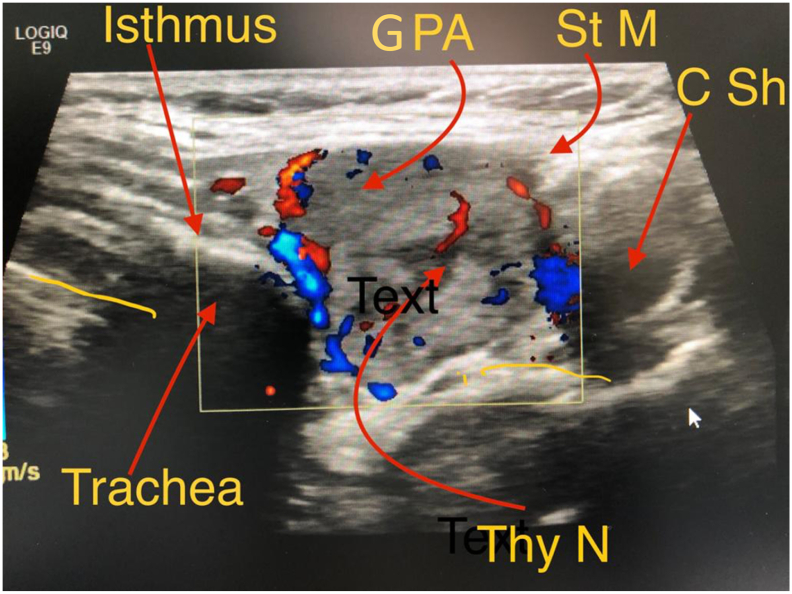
Ultrasound of thyroid nodule and parathyroid adenoma showing well-defined solid mass (21 × 18 × 36 mm) with increased vascularity in relation to the upper pole of the left thyroid lobe and lateral to the isthmus suggesting PA; and heterogeneous solid isoechoic nodule (23 × 16 × 38 mm) in the left thyroid lobe appearing wider than tall, with predominant peripheral vascularity suggesting a left thyroid nodule (GPA: giant parathyroid adenoma; St M: strap muscle; C Sh: carotid sheath; Thy N: thyroid nodule).
